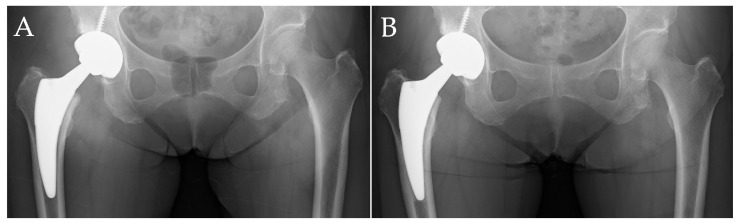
A representative case without evidence of stress shielding. Case of a 76-year-old female patient. (A) Anteroposterior radiograph of the hip immediately after surgery; (B) anteroposterior radiograph one year postoperatively. At one year postoperatively, no significant cortical bone thinning was observed, and there were no definitive indications of stress shielding.
